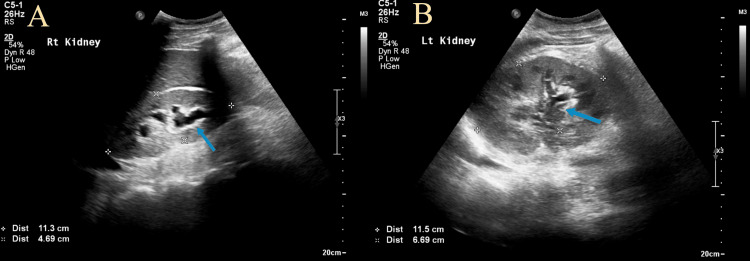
Ultrasound of the kidneys demonstrating bilateral hydronephrosis (blue arrows) with (A) the right kidney measuring 11.3 cm by 4.7 cm and (B) the left kidney measuring 11.5 cm by 6.7 cm.
